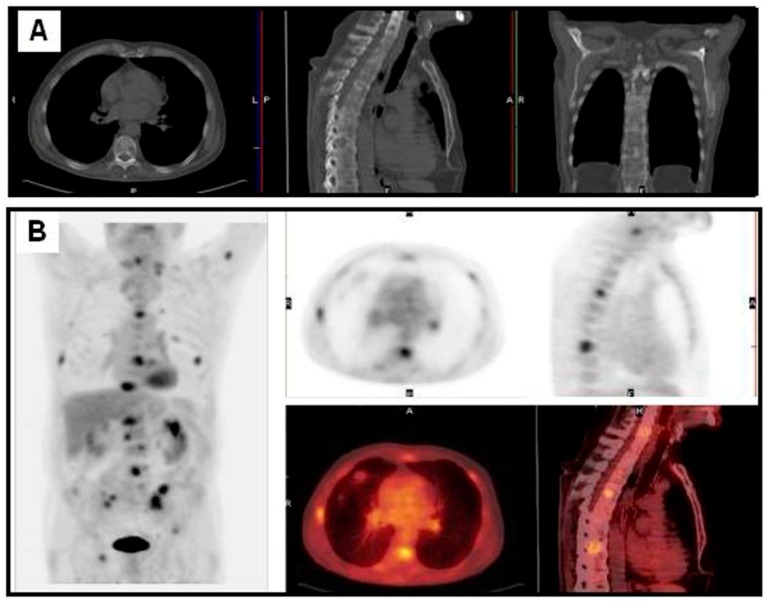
Patient with a NSCLC in the left superior lobe treated three years before with chemo-radiotherapy. A radiological exam showed sclerotic bone lesions (A); FDG PET/CT showed multiple bone, left supraclavicular lymph nodes and lung metastases (B).
